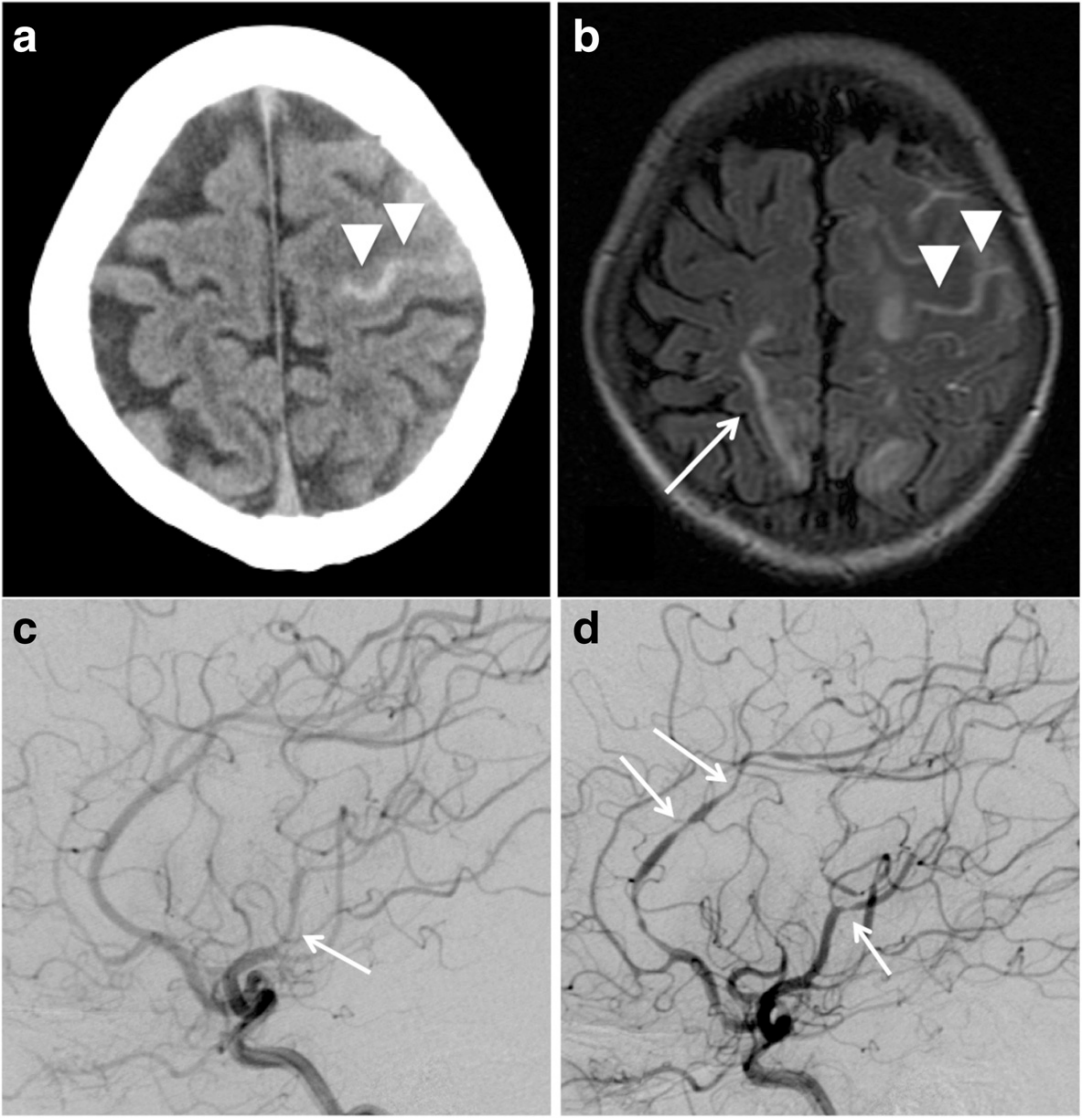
Neuroimaging findings of Case 1. a CT scan of the head shows a cortical subarachnoid hemorrhage along the left frontal sulci (*arrow heads*). b FLAIR sequence of the MRI shows the distribution of a subarachnoid hemorrhage (*arrow heads*) and hyperintense signal in the cortical-subcortical regions of the right parietal lobe (*arrow*). c Lateral view of the left internal carotid angiogram shows stenosis in the area of the peripheral middle cerebral artery. d Lateral view of the left internal carotid angiogram obtained ten days after onset shows diffuse segmental stenosis in the area of both the anterior and middle cerebral artery
